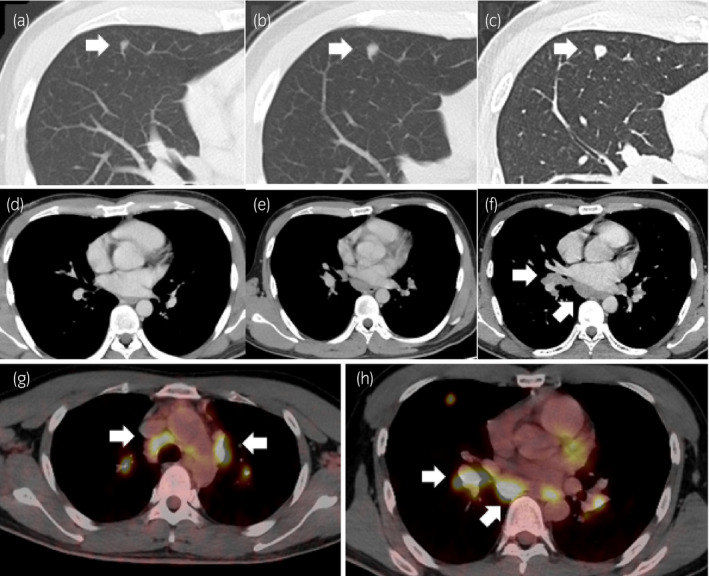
Imaging findings of a lung nodule with mediastinal lymphadenopathy of a patient with testicular seminoma after right orchiectomy. Progressing lung nodule at (a) 3, (b) 6, and (c) 9 months after orchiectomy. Development of mediastinal lymphadenopathy at (d) 3, (e) 6, and (f) 9 months after orchiectomy. PET/CT fusion images revealed accumulation of ^18^F‐labeled fluorodeoxyglucose in the lung nodule and mediastinal lymph nodes.
